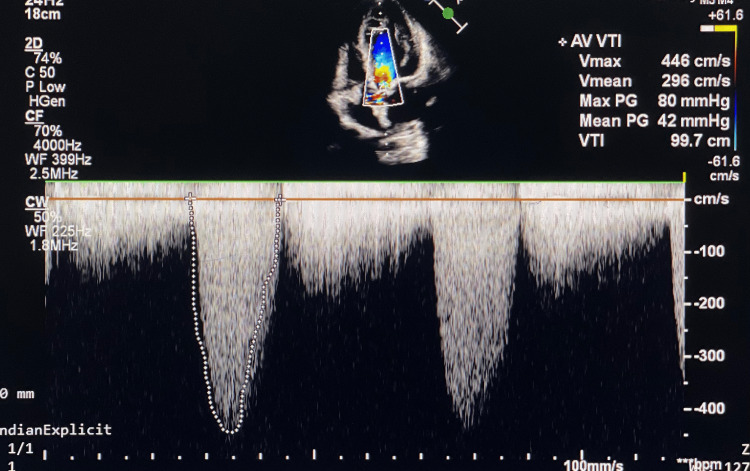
Severe aortic stenosis on 2D echocardiography 2D: two-dimensional Pulsed-wave Doppler echocardiogram on transthoracic, apical, five-chamber view showing AVmax of 446 cm/seconds with peak gradient across the aortic valve of 80 mm Hg and mean gradient of 42 mm Hg depicting severe aortic stenosis
